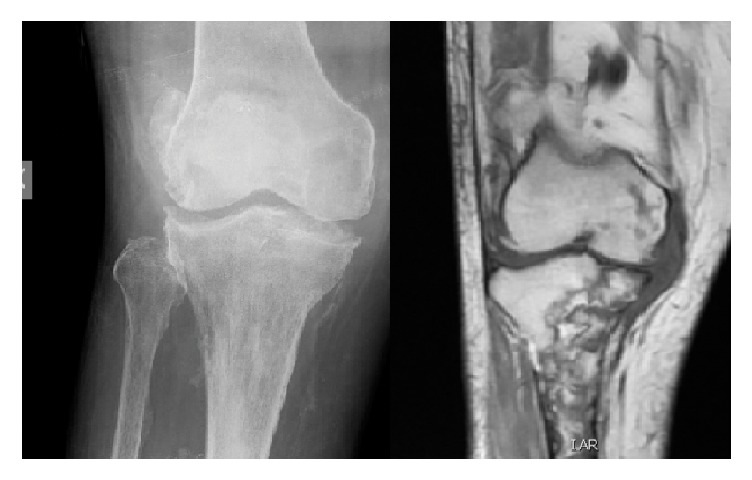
X-ray and MRI showing both knee septic arthritis and tibial and femur osteomyelitis.
